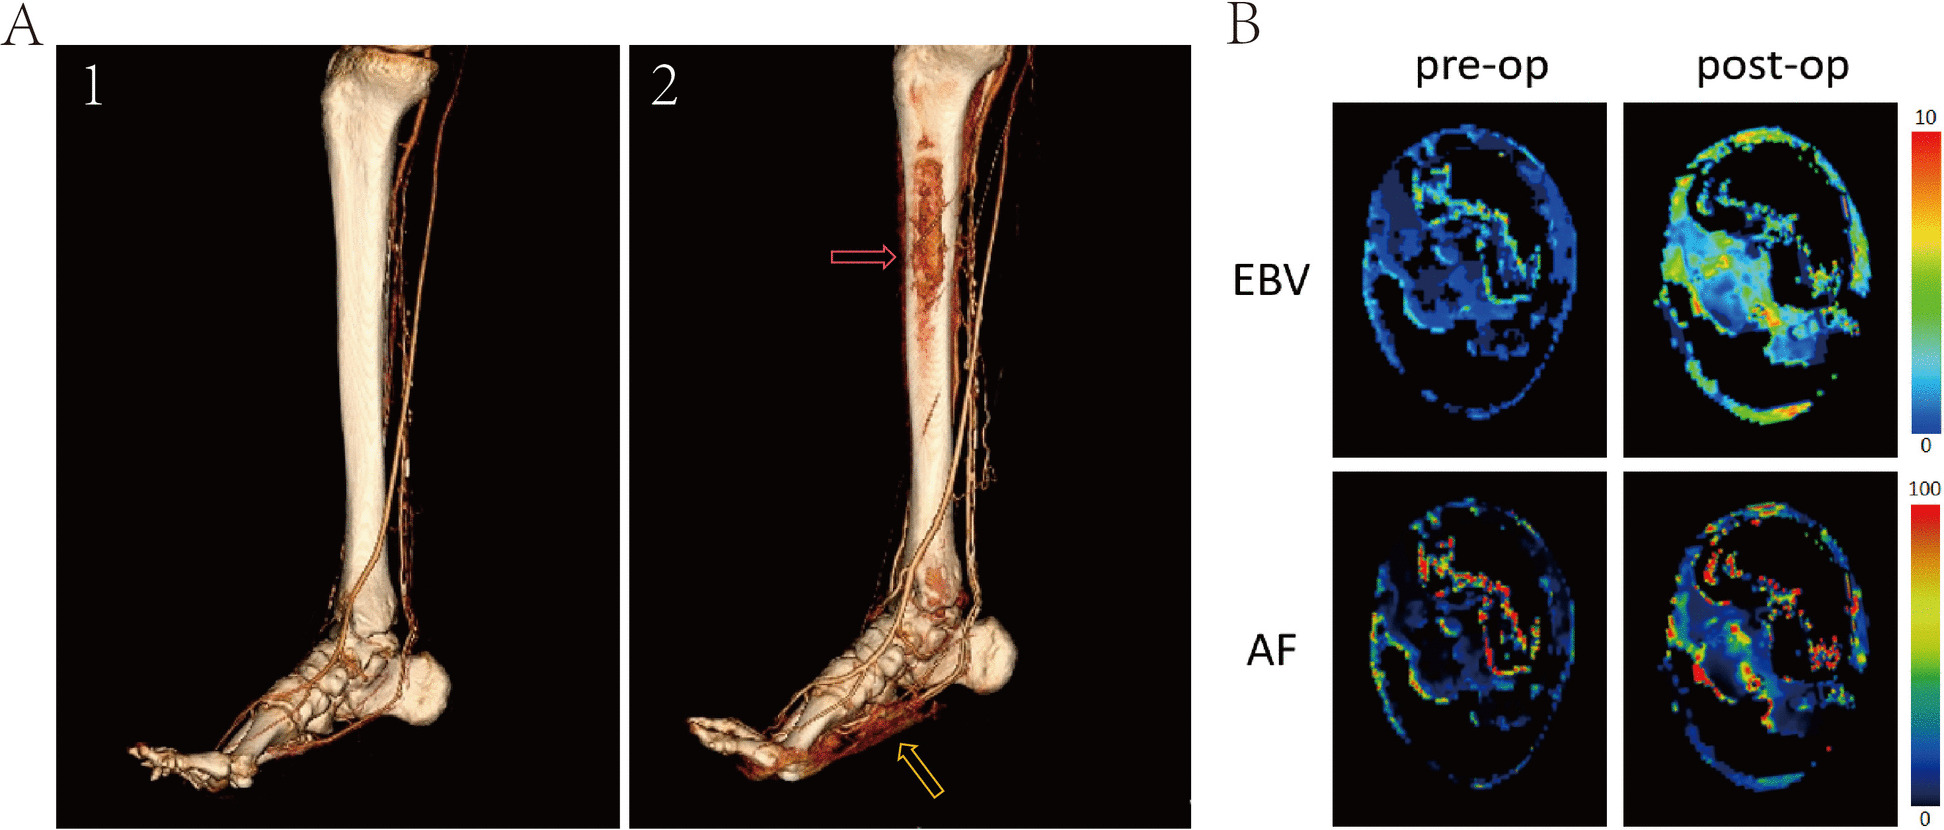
Pre- and postoperative lower limb imaging changes in patients. A Lower limb CTA. Postoperatively, dense microvascular networks (red arrow) can be seen at the surgical site on the medial aspect of the tibia, with increased vascular visualization (yellow arrow) in the foot. (1) Preoperative lower limb CTA. (2) Postoperative lower limb CTA after removal of the periosteal frame. B Axial CTP images of the foot. Postoperatively compared to preoperatively, EBV and AF significantly increased, with more complete foot visualization contours
